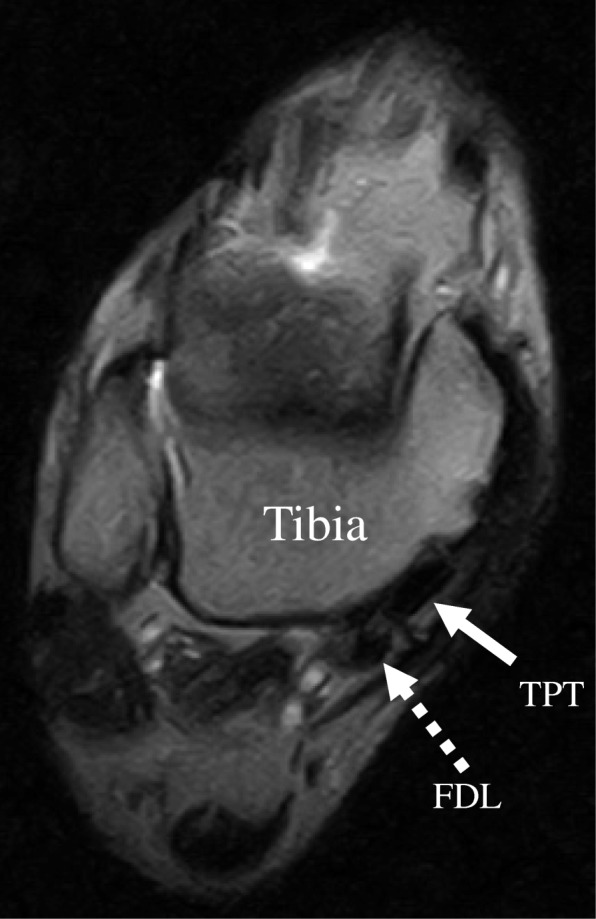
Latest 12-month postoperative magnetic resonance image shows the tibialis posterior tendon in a normal anatomical position. TPT (tibialis posterior tendon), FDL (flexor digitorum longus tendon)
